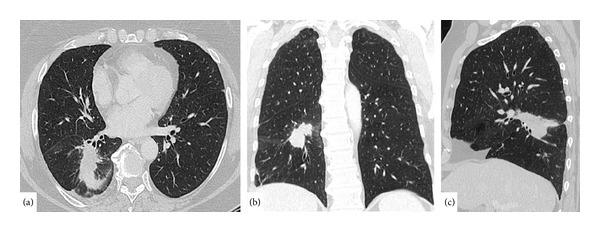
Axial, coronal, and sagittal CT images showing mass lesion in the inferior lobe of right lung.
